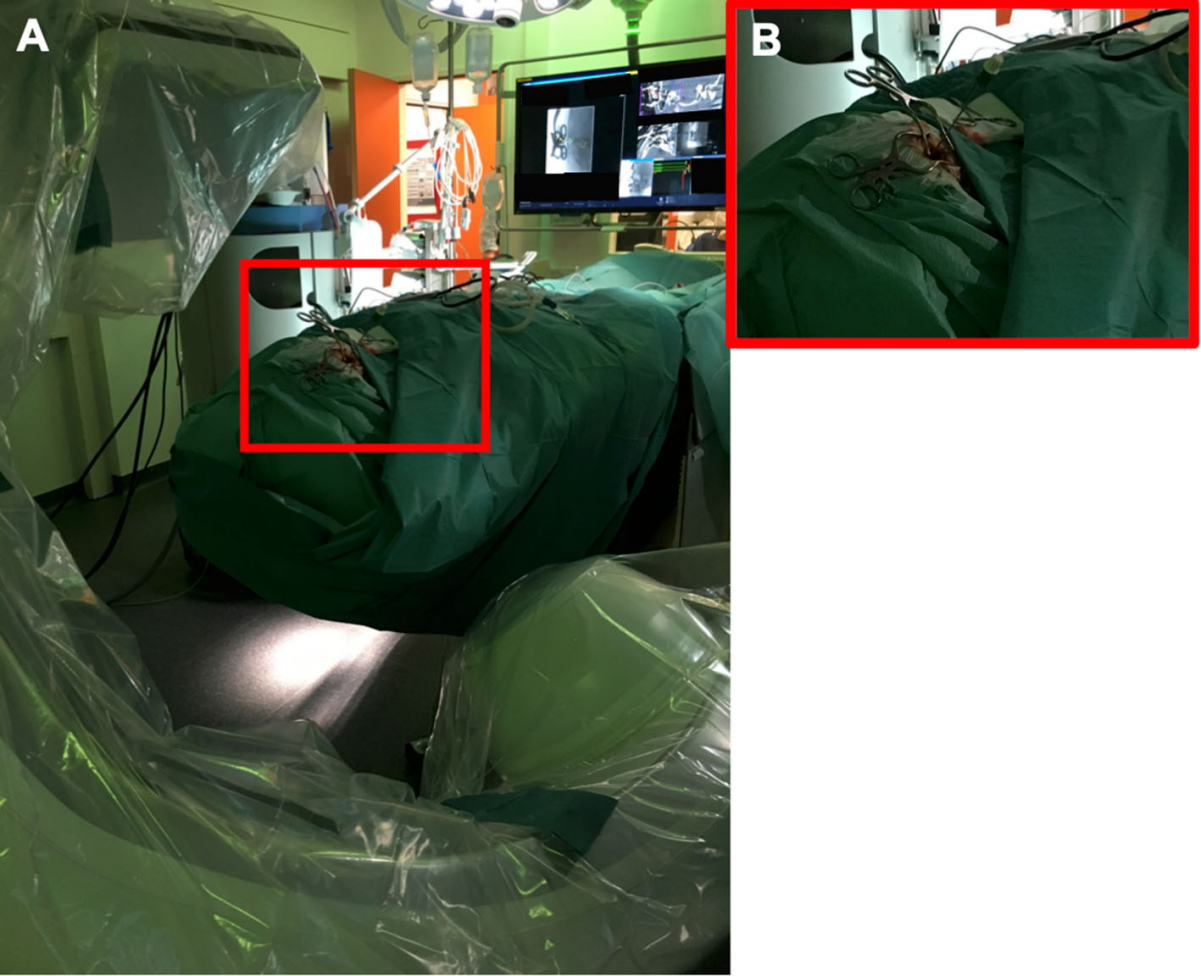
Surgical setup. Visualization of the surgical setup. A Operating field with mastoidectomy centered between detector (left upper corner) and X-ray tube (right lower corner). B enlarged view of the operating field
